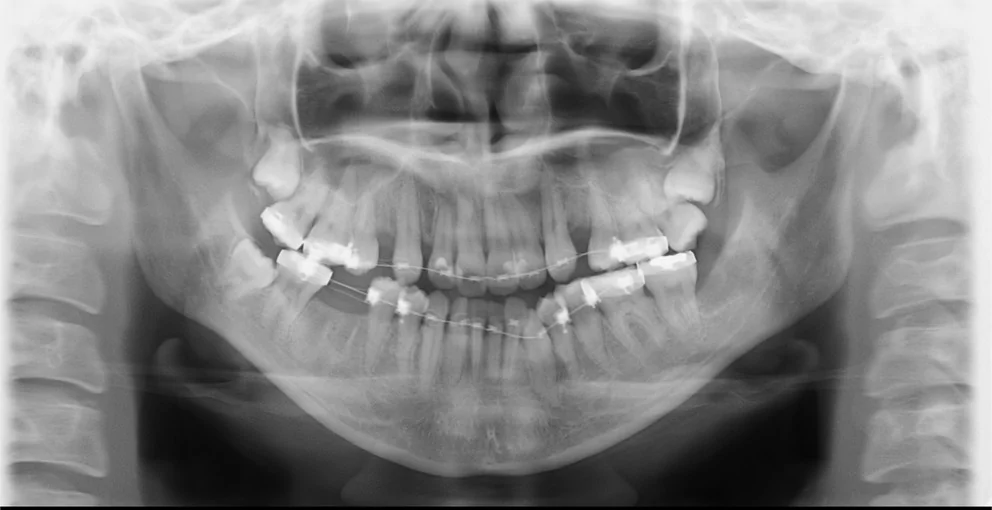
A panoramic radiograph image. The patient underwent extraction of teeth the mandibular right first molar, the mandibular left third molar and the maxillary right first premolar, the maxillary left first premolar at another dental practice and returned to our clinic for extraction of teeth the mandibular right third molar, the maxillary right third molar, the maxillary left third molar. Panoramic X‐ray taken during this visit on July 5, 2024. Again, enamel pearls cannot be confirmed at the maxillary left third molar site in the left maxillary sinus floor.
